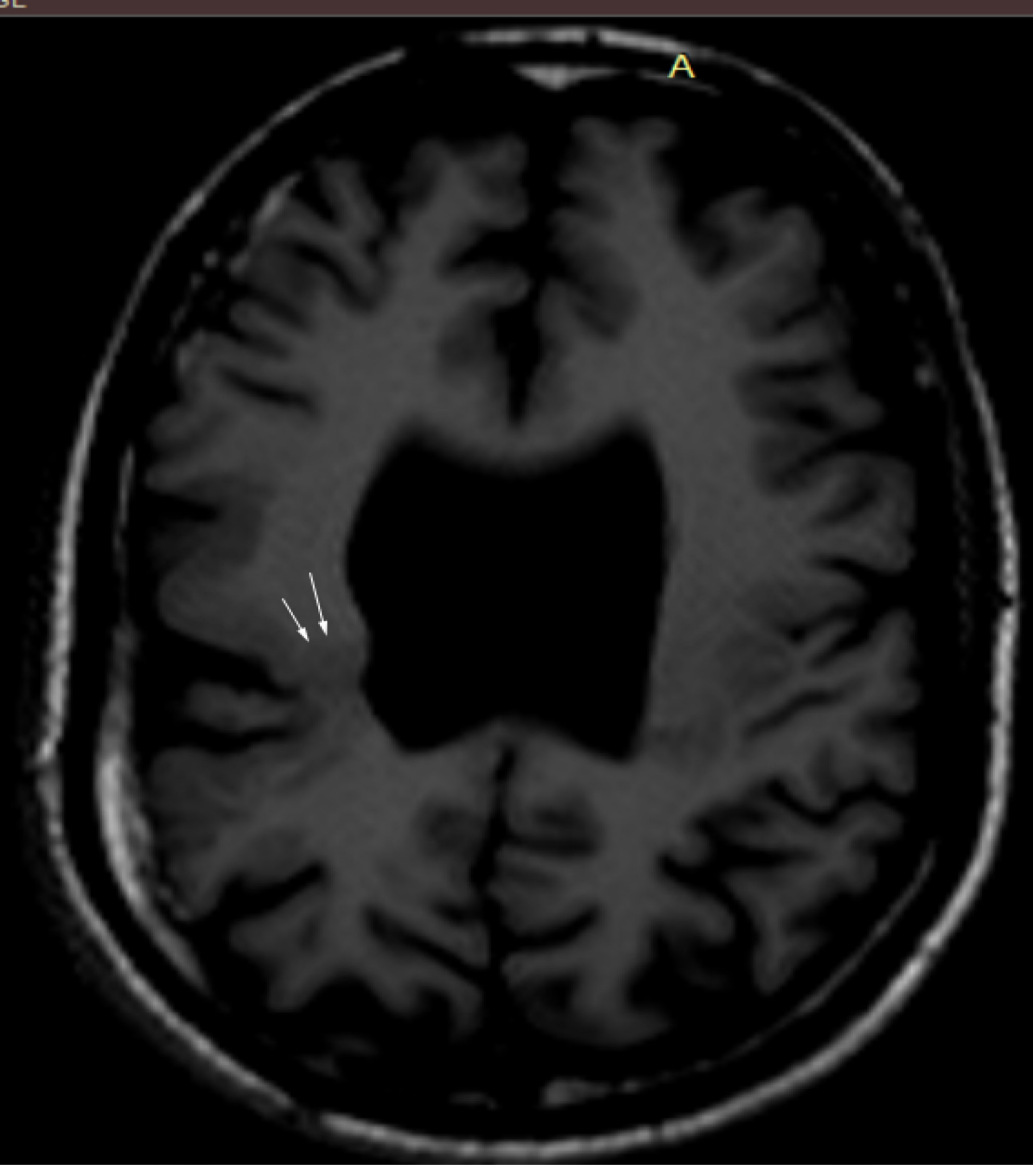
(At the level of lateral ventricle) T1W axial magnetic resonance imaging shows the abnormally located gray matter (white arrows) lining the T2 hyperintense line.
